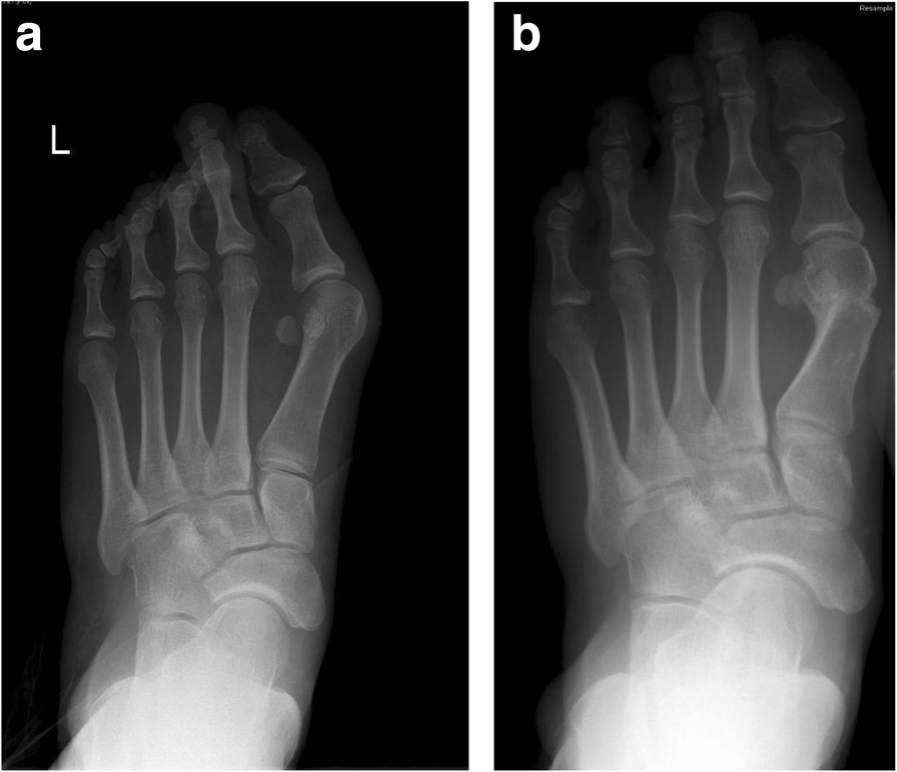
Anteroposterior radiographs of a patient’s left foot obtained before surgical correction of the hallux valgus (a, left panel) and 1 year after the surgery (b, right panel)
